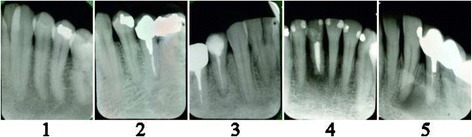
Grading of the Periapical Index: 1 = normal, 2 = bone structural changes, 3 = bone structural changes with signs of mineral loss, 4 = radiolucency, and 5 = radiolucency with features of exacerbation
